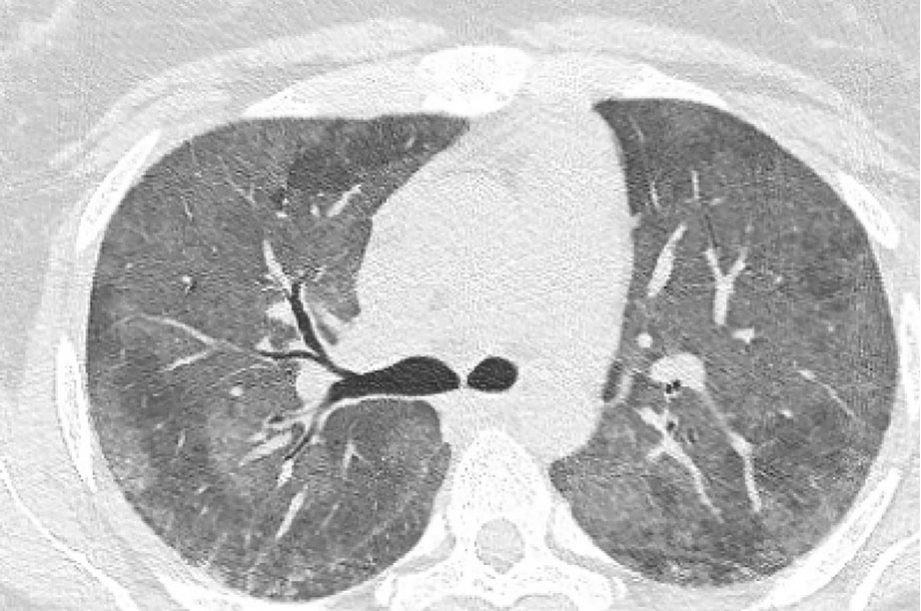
Computed tomography (CT) chest showing bilateral ground glass infiltrates in the upper lobes of the lung. Differing attenuation in various regions results in a mosaic attenuation.
